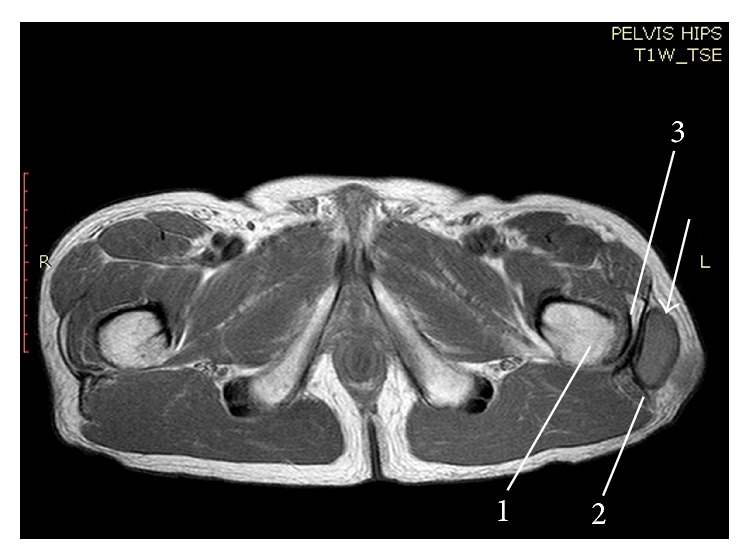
T1 weighted MRI image, depicting a cystic lesion (white arrow) located between the subcutaneous tissue and the underlying fascia of the iliotibial tract. There is no association of this lesion with the hip joint, findings consistent with a Morel-Lavallée lesion. (1) Greater trochanter, (2) iliotibial tract, and (3) the outer surface of the hip joint (L: left).
